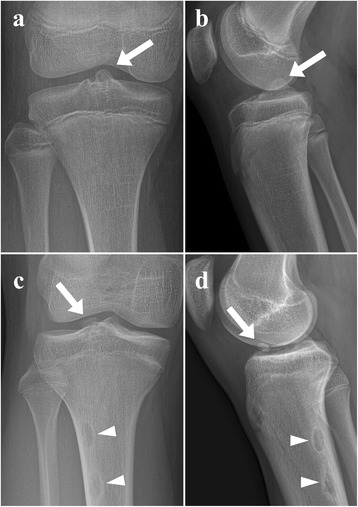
a, b Radiographs showing ledging (arrow), a prominent tibial eminence, possibly caused by malunion and overgrowth of the avulsed fragment. c, d In two patients, ossicles (arrow) were found above the tibial plateau. One patient had well demarcated intracortical osteolytic lesions (arrowhead) in the posterolateral aspect of proximal tibia
